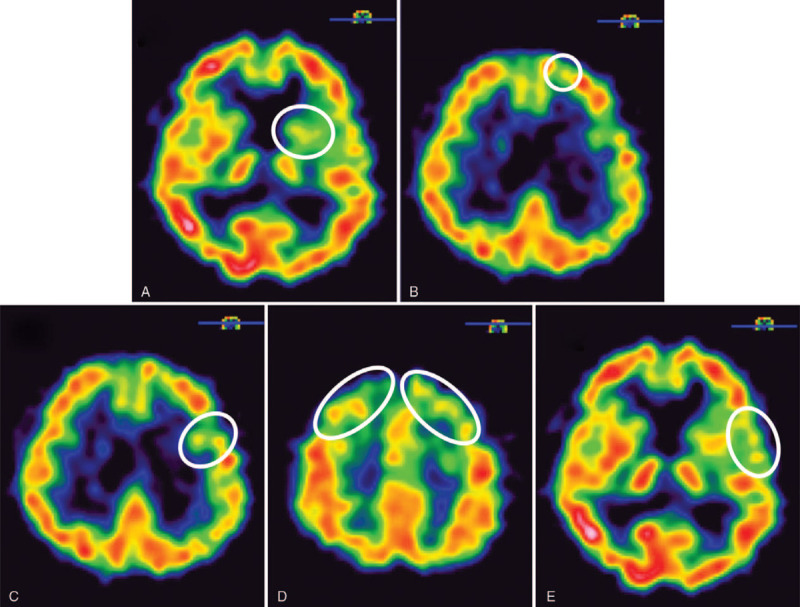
Axial Tc-99m ethylene cysteine diethyl ester brain single-photon emission computed tomography scan of the patient (Transverse view). The images reveal uneven cerebral perfusion with relative hypoperfusion (white circles) in the left parieto-occipital (4A), left frontal (4B), left parietal (4C), bilateral posterior frontal (4D), and bilateral, especially left, temporal (4E) regions. ECD = ethylene cysteine diethyl ester, SPECT = single-photon emission computed tomography.
